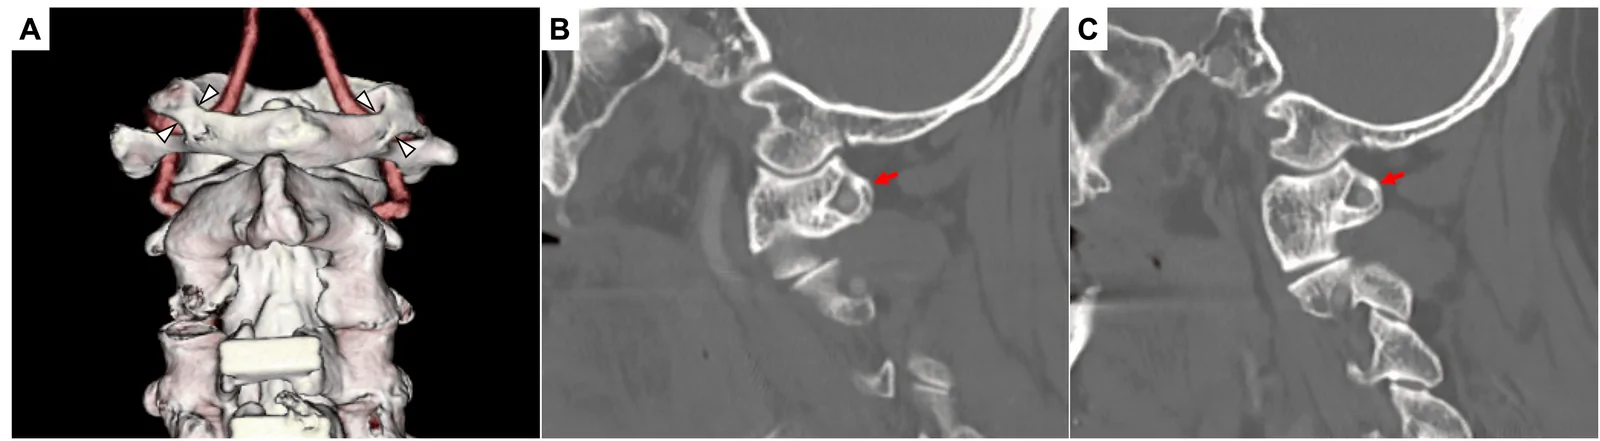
Three-dimensional CT angiography of bilateral complete ponticulus posticus. Posterior three-dimensional CT angiographic view (A) shows bilateral complete PP. The posterior arch appears broader because of bony bridges (white arrowheads) connecting the posterior arch to the cranial part of the lateral mass over the vertebral artery (VA). Parasagittal views of the preoperative CT scan (B, right side; C, left side) demonstrate a complete bony roof (red arrows) extending from the posterior arch to the lateral mass and surrounding the VA. Abbreviations: CT, computed tomography; PP, ponticulus posticus; VA, vertebral artery.
